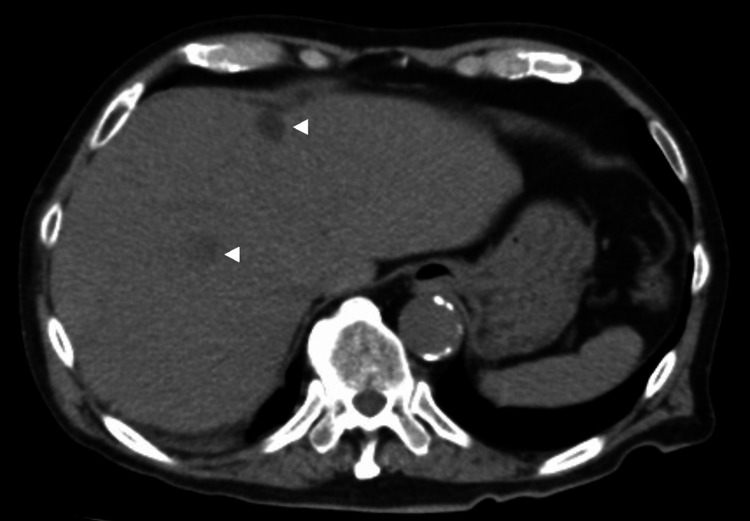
No abnormal liver lesions Abdominal plain CT: No abnormal findings were observed, except for a few hepatic cysts (white triangle).
